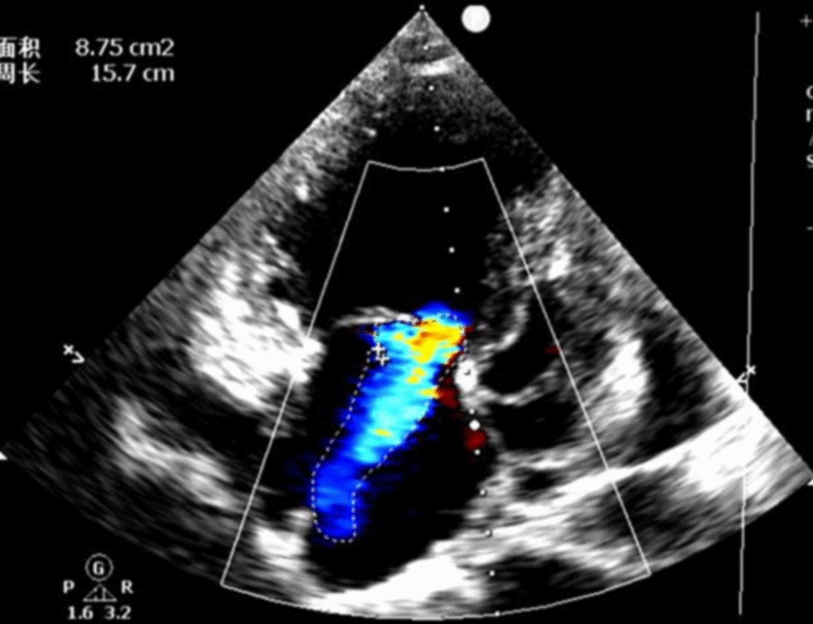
Severe tricuspid regurgitation on color flow Doppler
